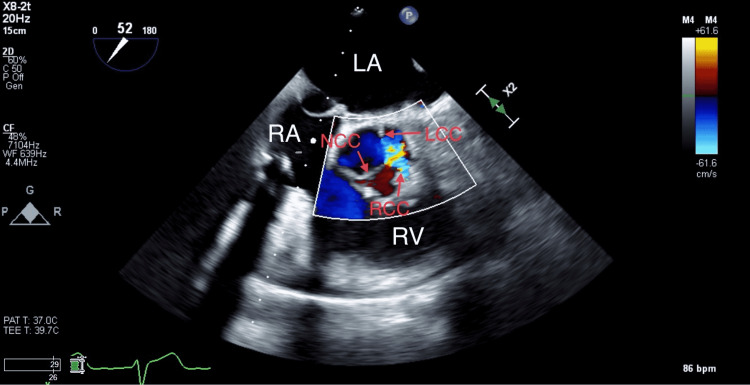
Mid-esophageal TEE aortic valve short-axis view showing aortic insufficiency due to aortic annulus entrapment. TEE: transesophageal echocardiogram, NCC: noncoronary cusp, LCC: left coronary cusp, RCC: right coronary cusp, LA: left atrium, RA: right atrium, and RV: right ventricle.
